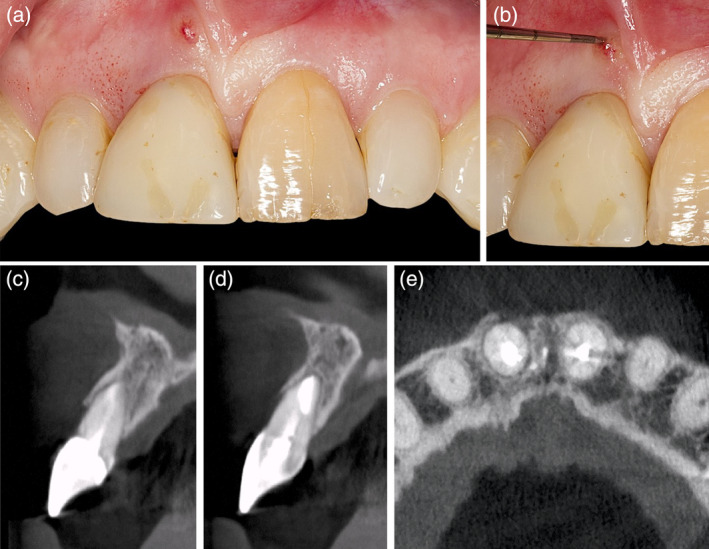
(a) and (b) clinical case with an endodontically treated but failing tooth in position #11 with a buccal fistula; (c)–(e) the computed tomography confirmed the buccal bone resorption in the region of the fistula
